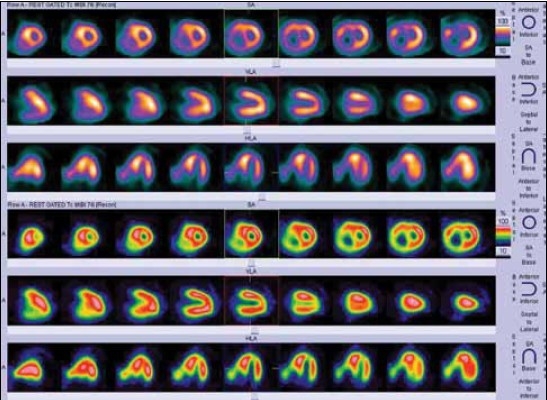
Rest SPECT images of an uncorrected tetrology of Fallot patient with biventricular dysfunction. Images show right ventricular enlargement, hypertrophy, and associated septal hypertrophy. There are no perfusion defects in LV myocardial segments at rest
